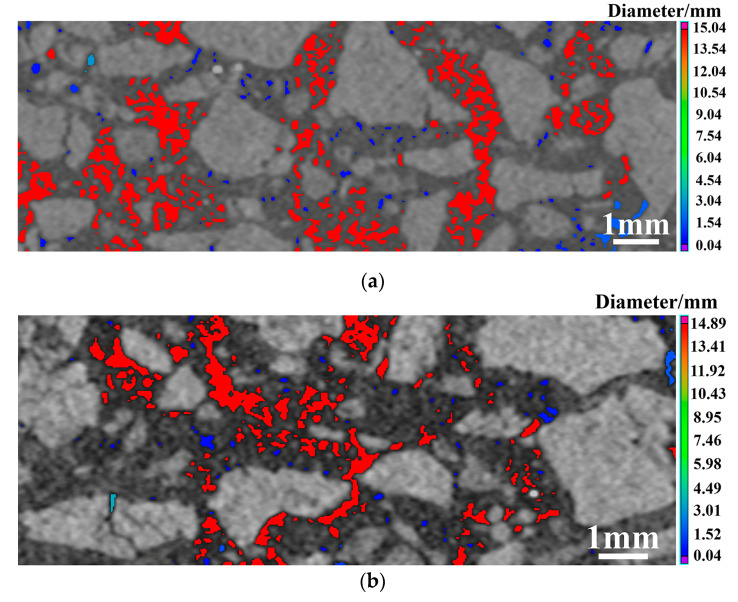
The CT images of the intact layer in the samples without (a) and with (b) Mg-sialon.
